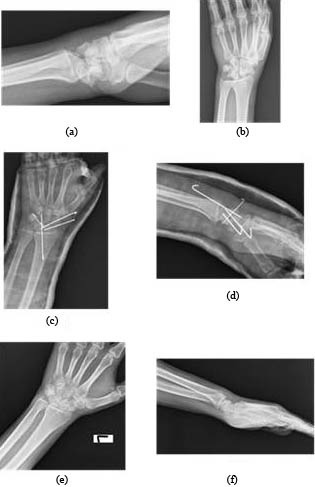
The radiographs of patient 4, a 32-year-old man who
